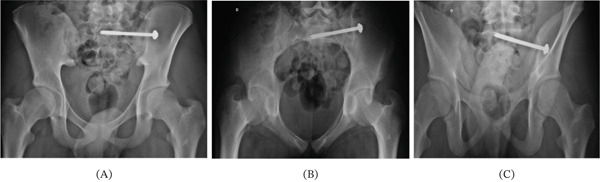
Postoperative radiographs. Postoperative pelvic radiographs in the (A) anteroposterior (AP), (B) inlet, and (C) outlet views.
